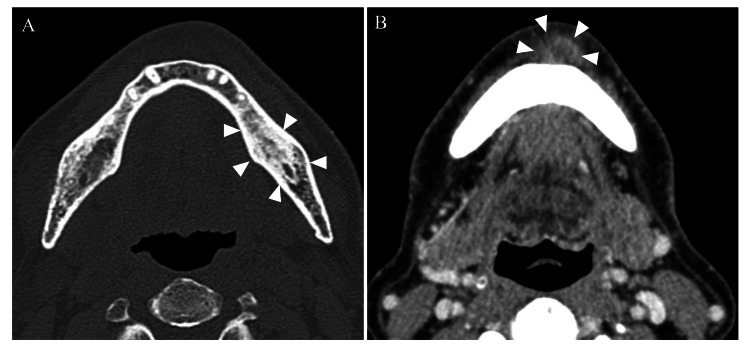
Contrast-enhanced CT photograph A: bone mode; B: contrast-enhanced mode Osteosclerosis was observed in the left side of the mandible, equivalent to 37, and a relatively well-defined, oval-shaped area showing a contrast effect at the margins was observed in the left side of the mandible.
